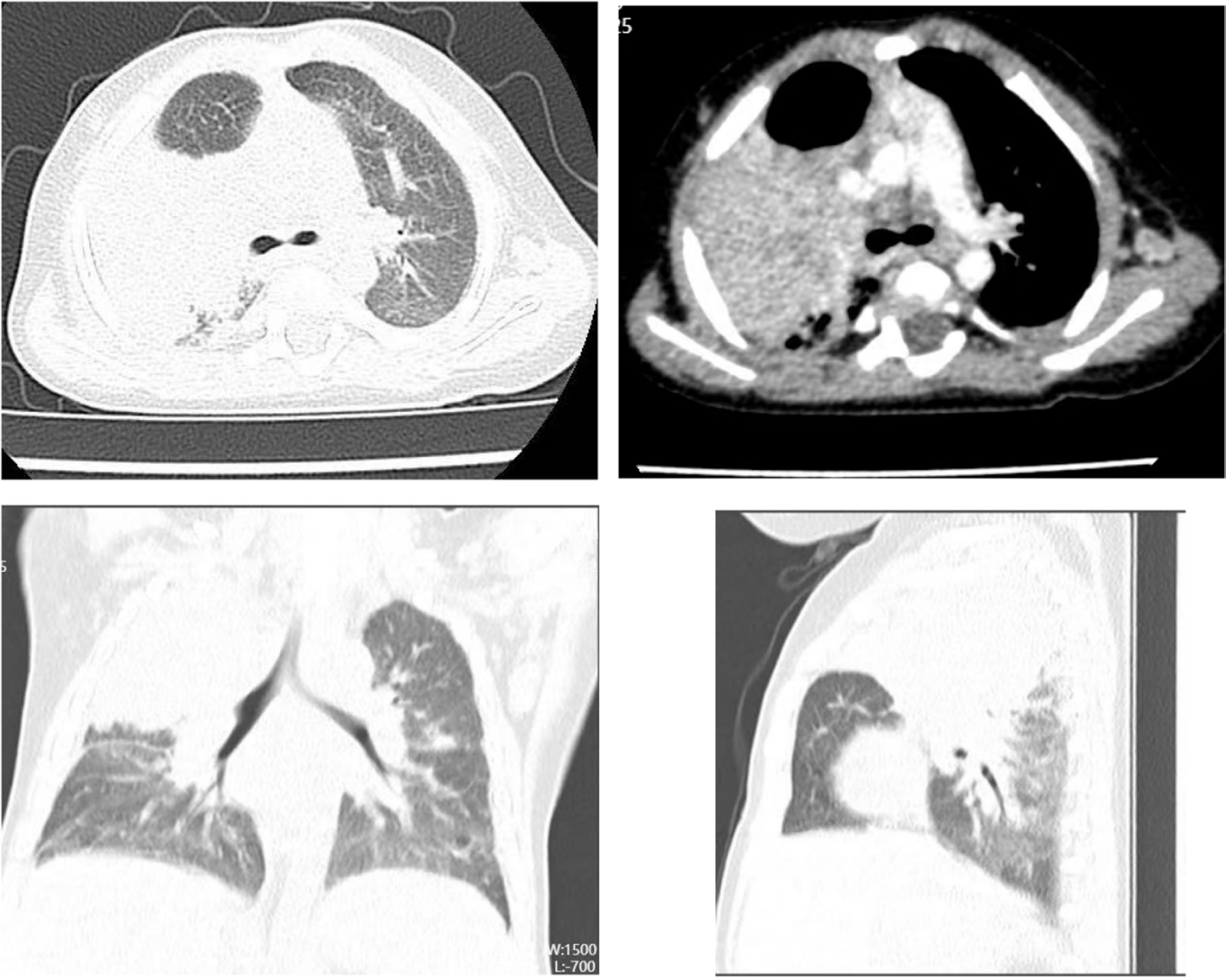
HRCT showing consolidation in the right upper lobe and multiple enlarged lymph nodes in the right hilum and mediastinum, as well as in the left axilla
